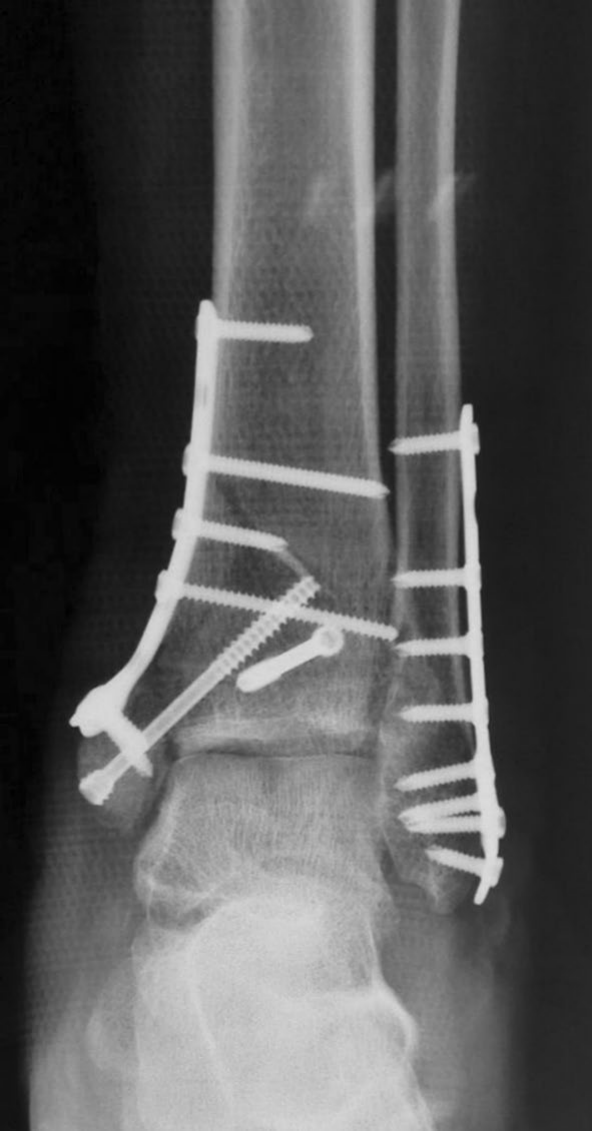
X-ray showed that inferior segments of right tibia and fibula had good reduction and the joint surface also recovered well after the surgery
